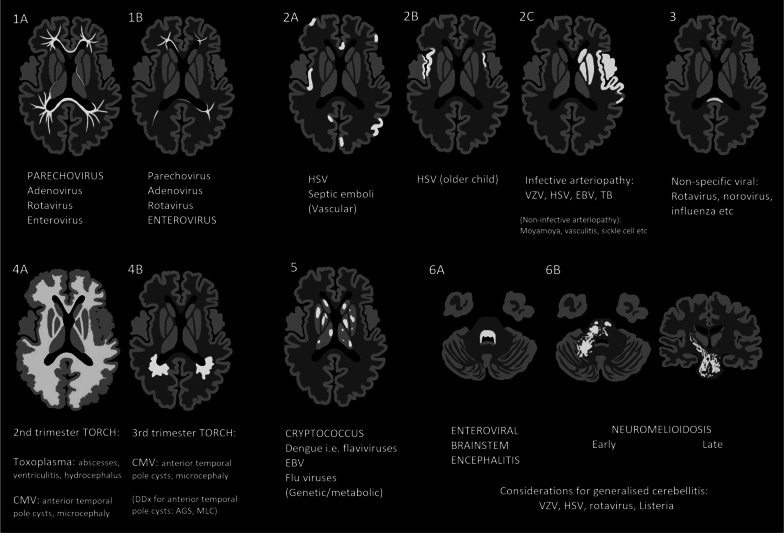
MRI patterns of paediatric brain infections, with differentials relevant to the WA environment. A summary of the key MRI patterns and their sub-patterns as discussed in this review, with the causative pathogens tailored to the WA environment
